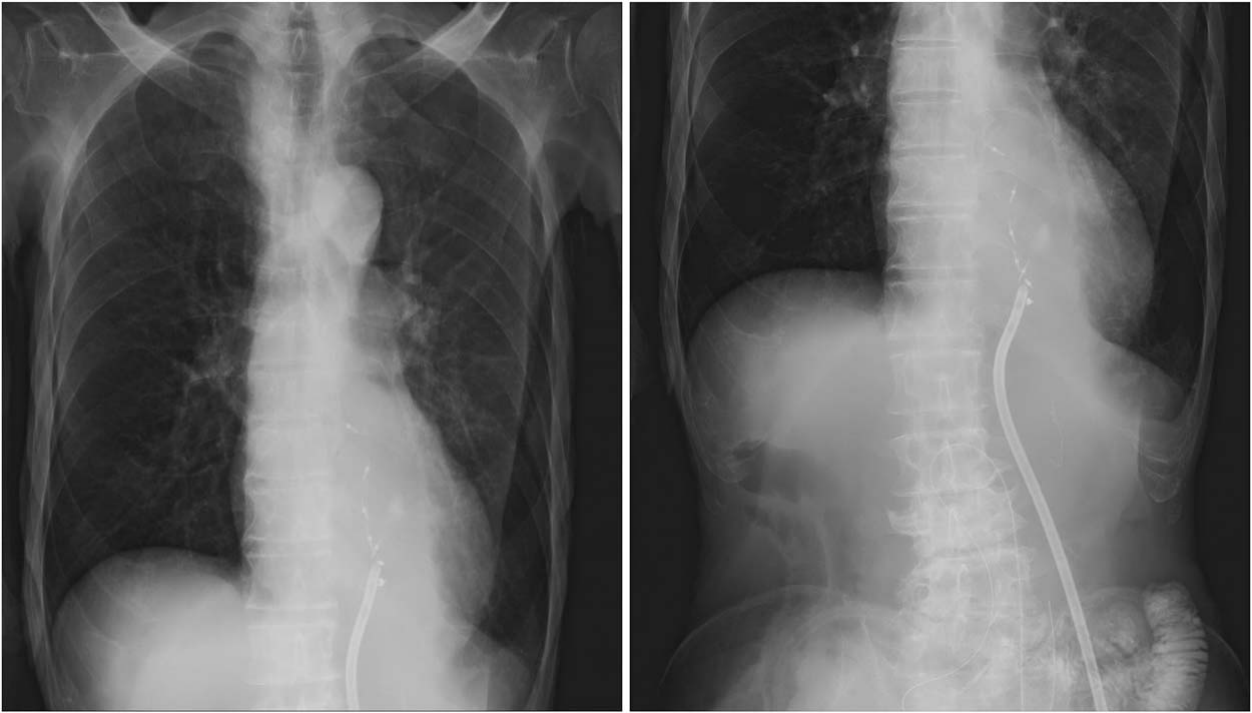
Drain repositioning. Chest radiograph and contrast study showing drain tip repositioning into the mediastinum.
